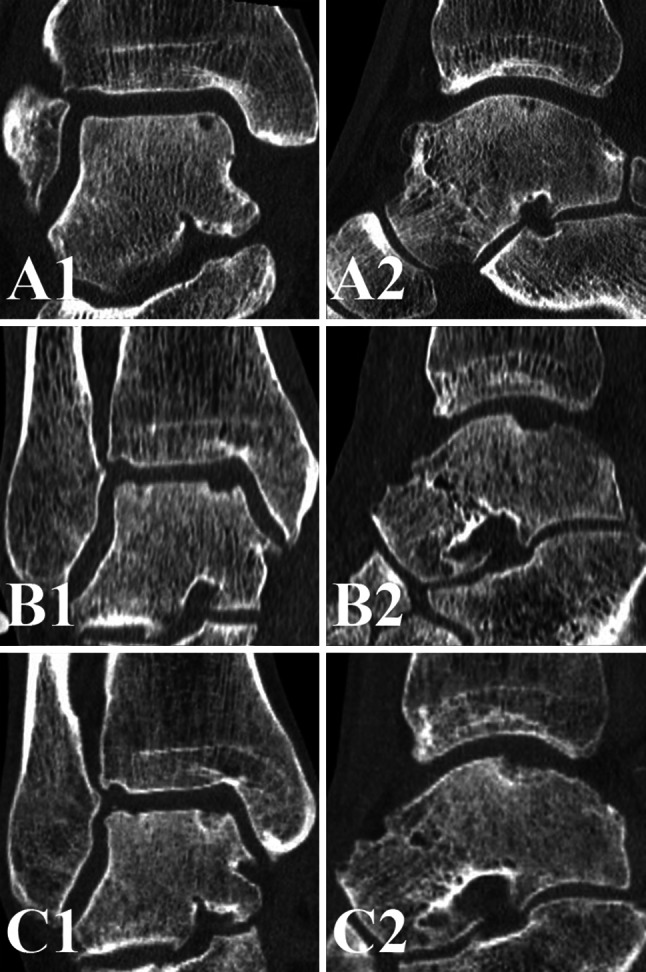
a Preoperative coronal (A1) and sagittal (A2) CT scans of a *right* ankle show a cystic OCD of the *medial* talar dome, b the two weeks postoperative coronal (B1) and sagittal (B2) CT scans show an increased defect size after technically successful debridement and microfracture, c at 1-year follow-up, the defect size decreased and the level of subchondral bone plate was almost flush [coronal CT scan (C1); sagittal CT scan (C2)]
